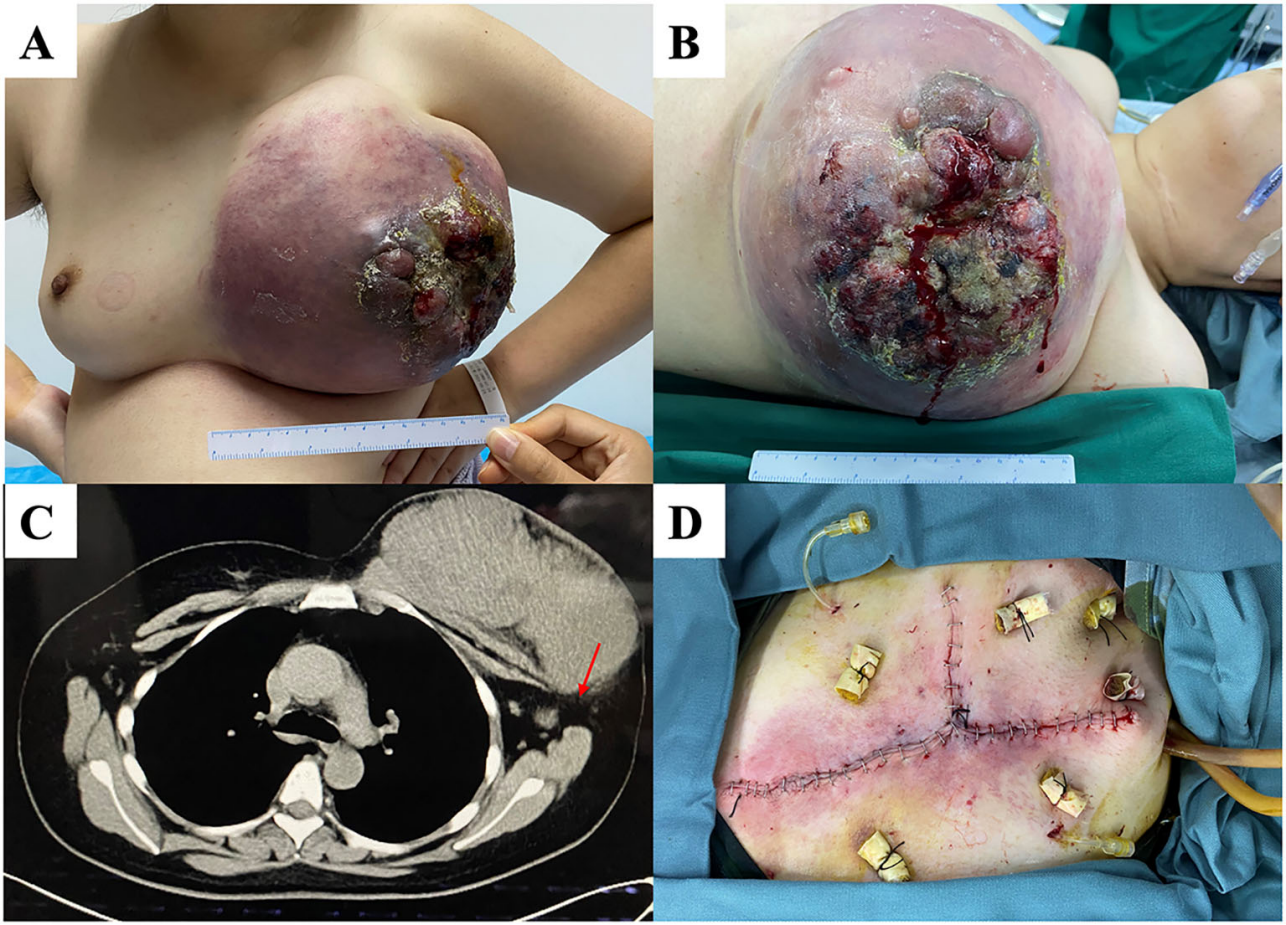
(A, B) A patient with a huge tumor occupying the whole left breast with a broken surface. (C) Chest computed tomography (CT) showing that the is closely tumor adhered to partially invading the chest wall. Enlarged lymph nodes (red arrow) are present. (D) We performed modified radical mastectomy, preserving as much of the normal skin tissue as possible.
